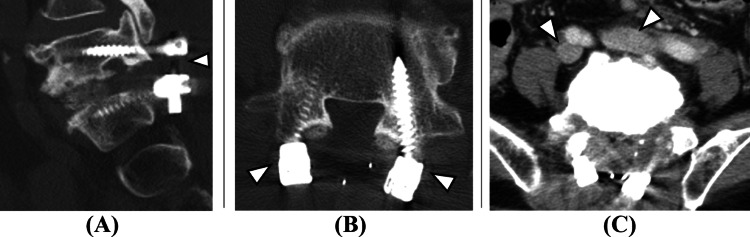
Immediate postoperative lumbar CT. (A) Sagittal, (B) axial, and (C) contrast-enhanced axial view. L5/S1 is stabilized with pedicle screws (white arrowheads, A and B). In the contrast-enhanced axial image at the L5/S1 disc level, the bilateral common iliac veins are seen adjacent to the ventral aspect of the disc. No significant hematoma formation is noted.
